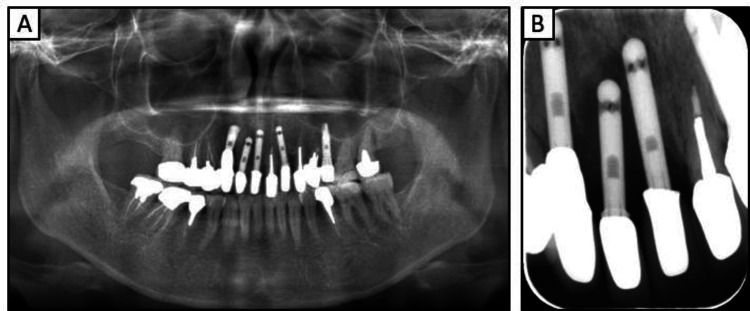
Preoperative radiographs obtained at the patient’s initial visit (A)  Panoramic radiograph (B)  Radiograph of the right anterior maxilla
